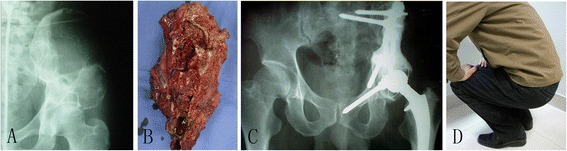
Case presentation of a 34-year-old man who mainly complained about pain in the left hip area for 5 months which was aggravated over the previous 1 week. a Osteolytic lesions can be observed in the left ilium and periacetabular region on the plain film. b The tumor was resected widely, and the cut line was at the normal bone. c A plain film at 2 years after operation shows no recurrence. d The patient has good function without any discomfort in the last follow-up
